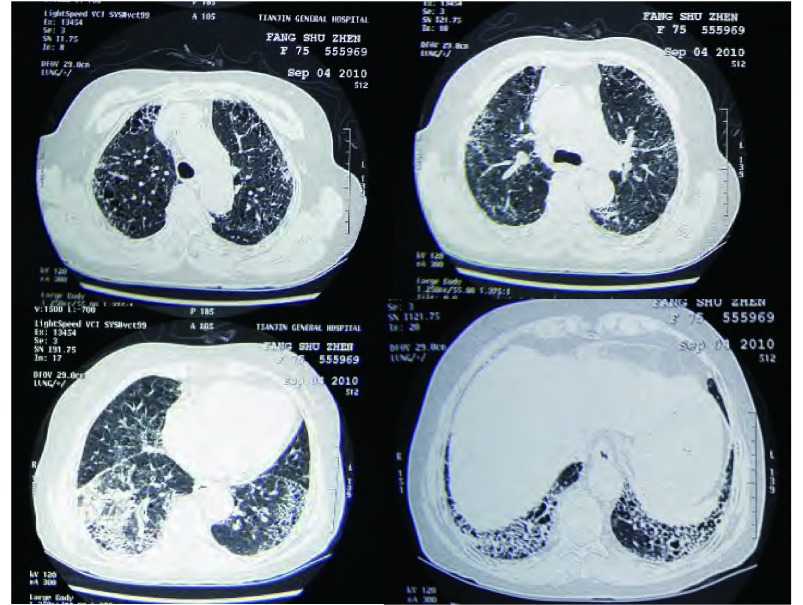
胸部HRCT：双上肺气肿；两肺间质炎症和间质纤维化改变，以两下肺外周区为著，呈蜂窝状改变，右下叶实变影。 HRCT of thorax: Emphysema existed in the upper lobes of the lungs, consolidation in the right lower lobe, marked coarse reticular opacities, traction bronchiecatsis, and honeycomb cyst in the basilar and peripheral (subpleural) regions of the lungs.
